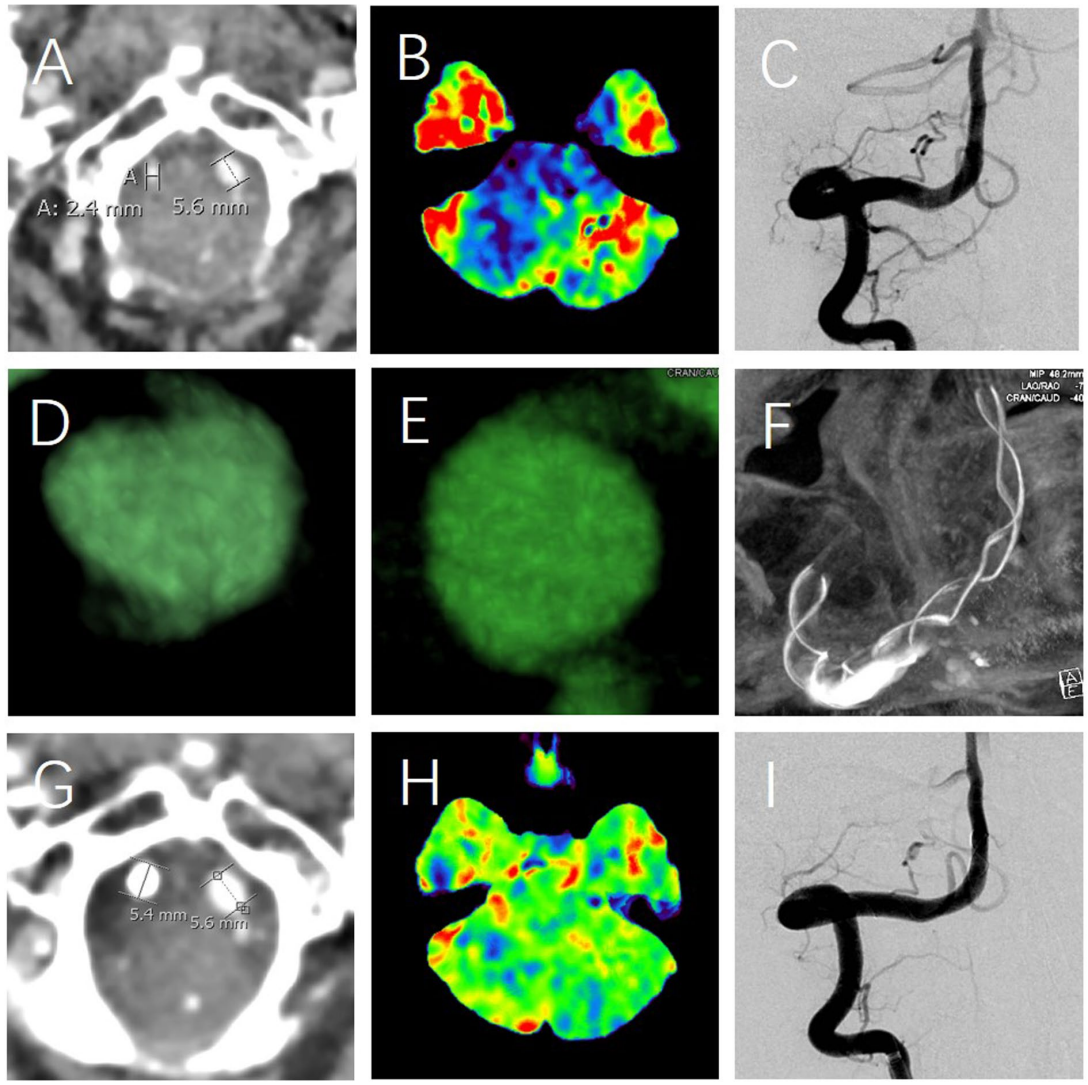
The diagnosis and treatment of multi-regional sequential pain syndrome. The first abnormality observed is a smaller right vertebral artery (A), coupled with a relatively better perfusion of posterior circulation (blue, B). DCE-CT identified the right CVAD, characterized by the normal vascular morphology (C) and abnormal intimal flaps (white arrow, D,E), which was subsequently addressed using a stent (F); The second significant abnormality observed was as follows: despite DSA showing no change in the morphological appearance of the vertebral artery (C vs. I), there were considerable changes detected in the vascular status (A vs. G) and perfusion (B vs. H) levels before and after the treatment. Specifically, the vascular status (A) and perfusion (B) exhibited marked differences when juxtaposed with their post-treatment counterparts in G and H, respectively.
